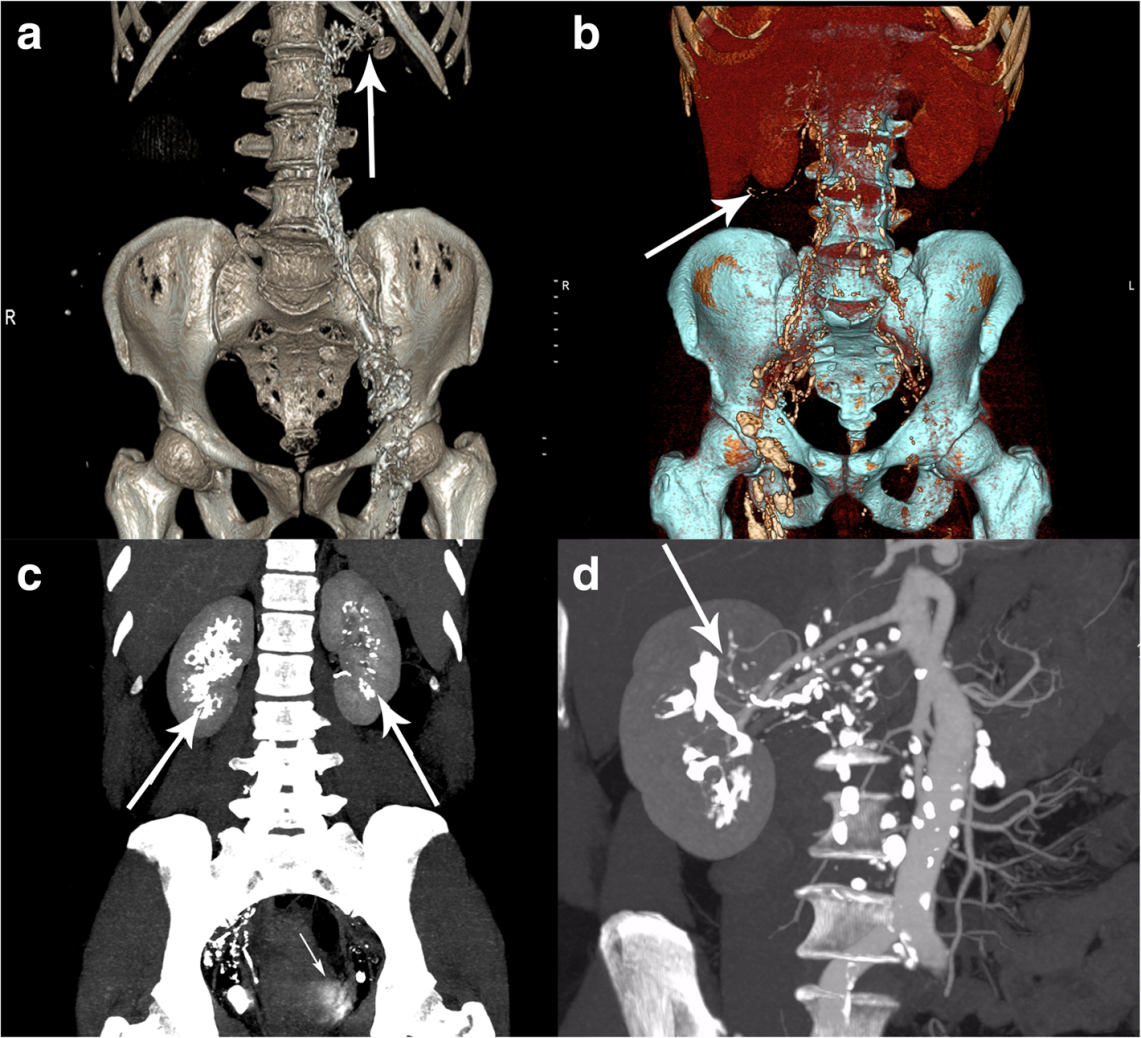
VR of CT data showing (a) the distribution of lymphatic vessels around the left renal artery and vein. b Chyle leaks in right renal fascia. MIP of CT data showing (c) Bilateral lymphatic leakage of the renal pelvis, and reflux of contrast agent into the bladder and (d) right renal lymphatic leakage and lymphatic vessel lesions adjacent to the renal vein
